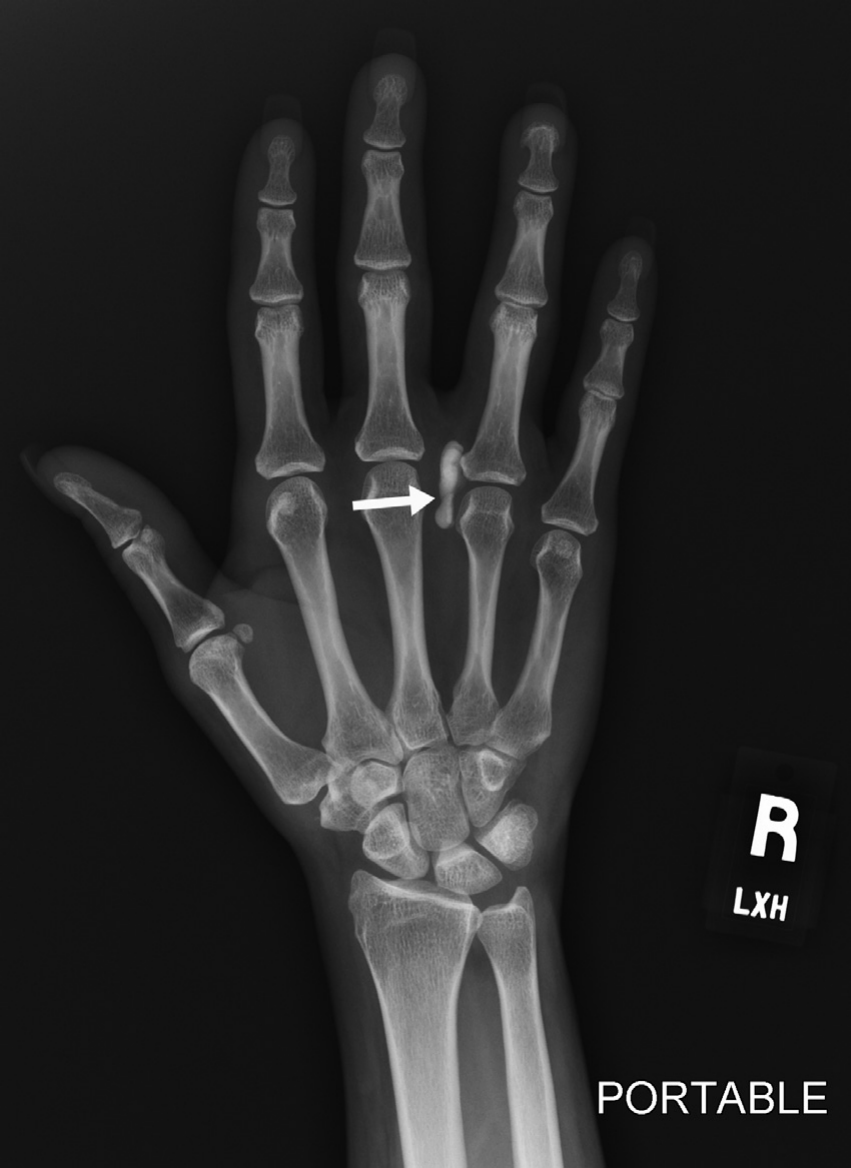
Frontal radiograph of the right hand demonstrates longitudinal calcification in between the third and fourth MCP joint (arrow), with surrounding soft tissue swelling. These findings are compatible with calcific periarthritis.
